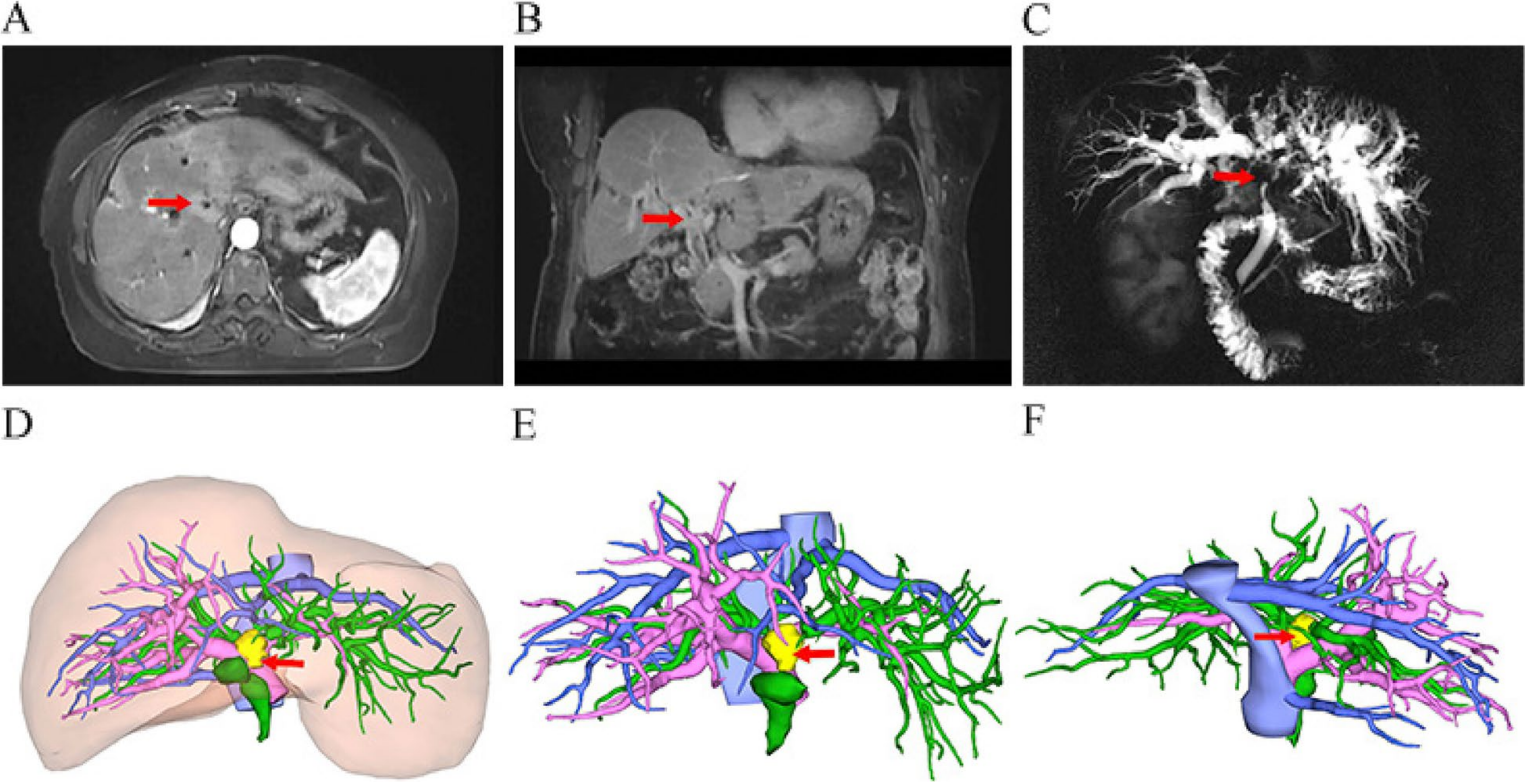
A Location of the tumor on the MRI cross-section. B Coronal MRI showing the shape of the tumor. C MRCP showing the intrahepatic and extrahepatic biliary systems. D Three-dimensional reconstruction showing the relationship between the liver and the biliary system, blood vessels, and tumor. E Frontal three-dimensional reconstruction showing the relationship between the biliary system, blood vessels, and tumor. F Three-dimensional reconstruction of the relationship between the biliary system, blood vessels, and tumor, as shown in lateral view
